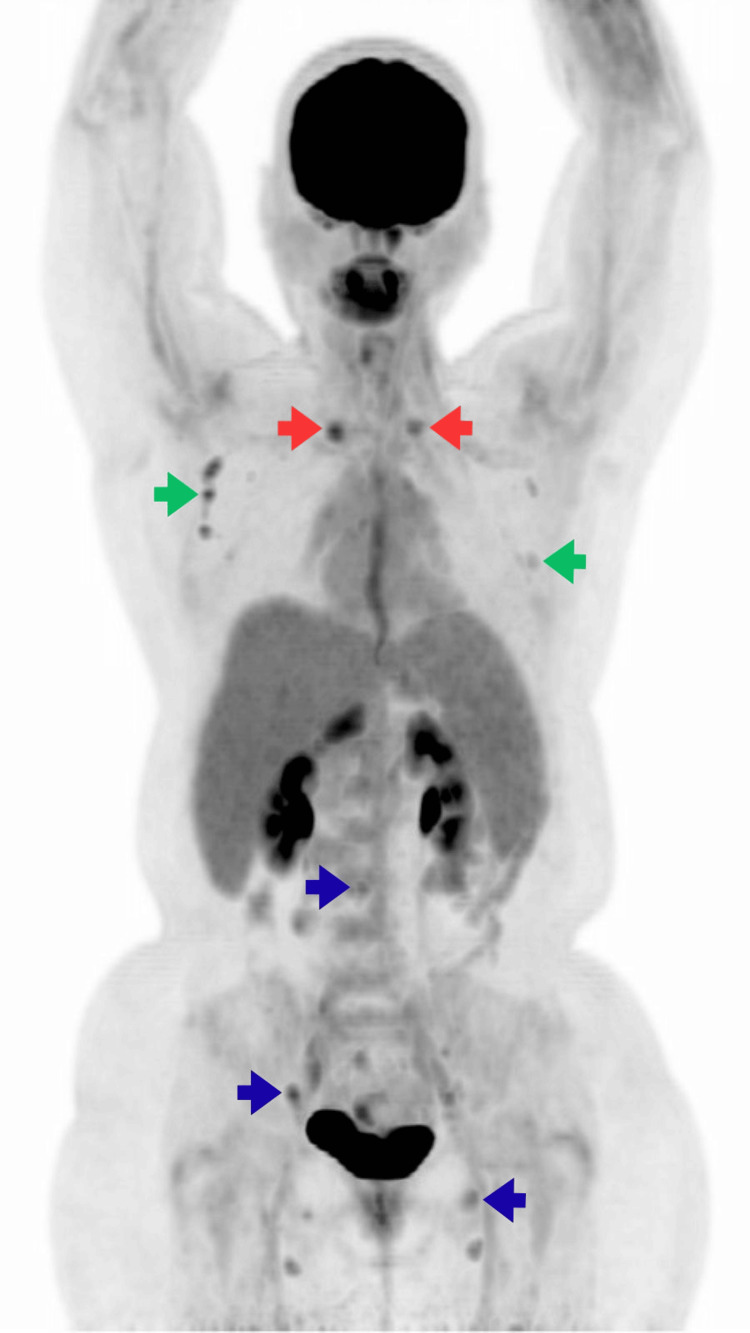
Maximum intensity projection (MIP) of the whole-body ¹⁸F-FDG PET/CT. Hypermetabolic cervical lymph nodes (red arrow) and osseous lesions are visualized (blue arrow). Bilateral axillary lymph nodes with a reactive appearance are also noted (green arrow). ¹⁸F-FDG: 18F-fluorodeoxyglucose; PET/CT: positron emission tomography/computed tomography
